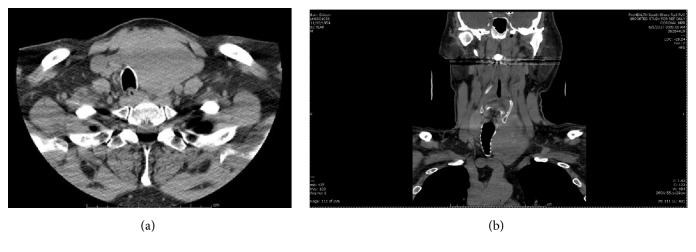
Preoperative neck CT without contrast, demonstrating a large left thyroid lobe mass with displacement of the trachea, mild compression, and early substernal extension; representative axial (a) and coronal views (b).
